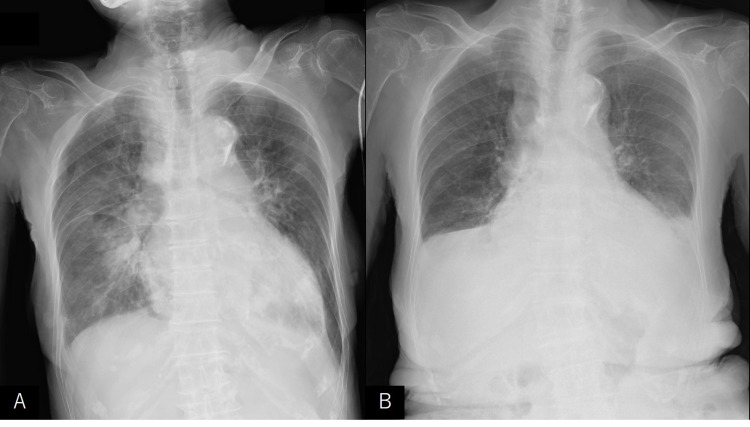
(A) Initial x-ray shows diffuse alveolar infiltrates throughout both lungs. (B) The pulmonary edema also improved on the sixth day of hospitalization. On the contrary, pleural effusion remained.
